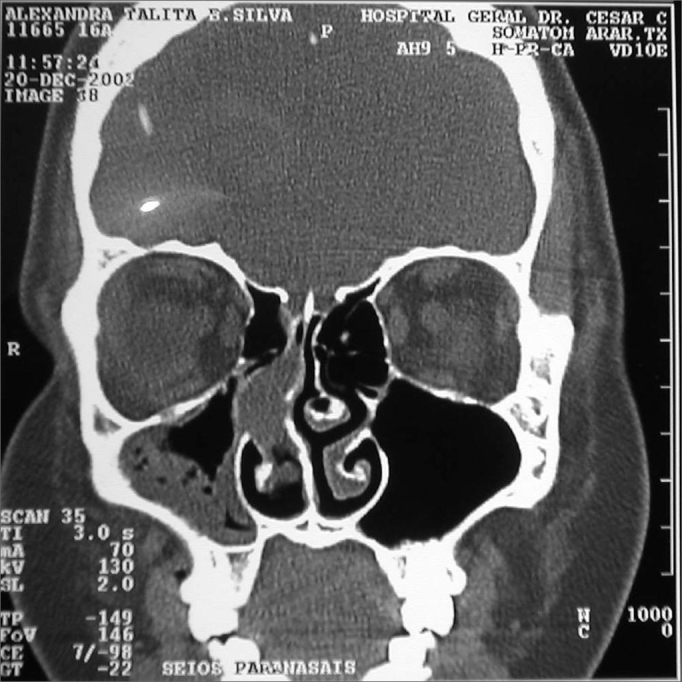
CT scan. Coronal view of a Stesioneuroblastoma (case 1).
